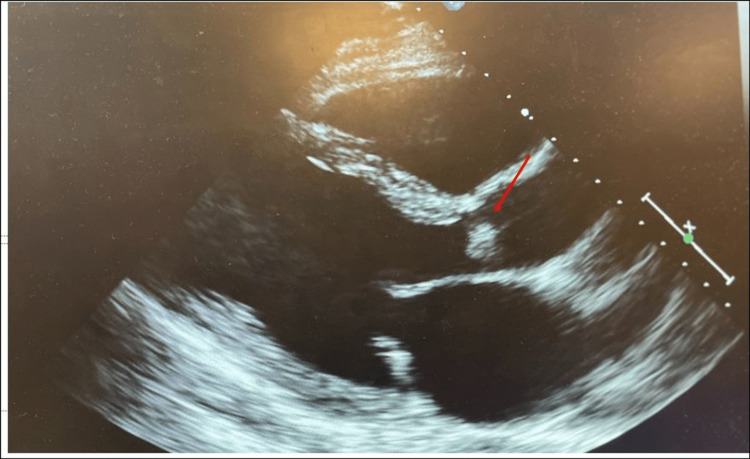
Transthoracic echocardiographic view: transverse long-axis view showing the aortic valve with a mobile echo density. Transthoracic echocardiogram depicting a mobile echo density (red arrow) attached to the aortic valve in the transverse long-axis view.
